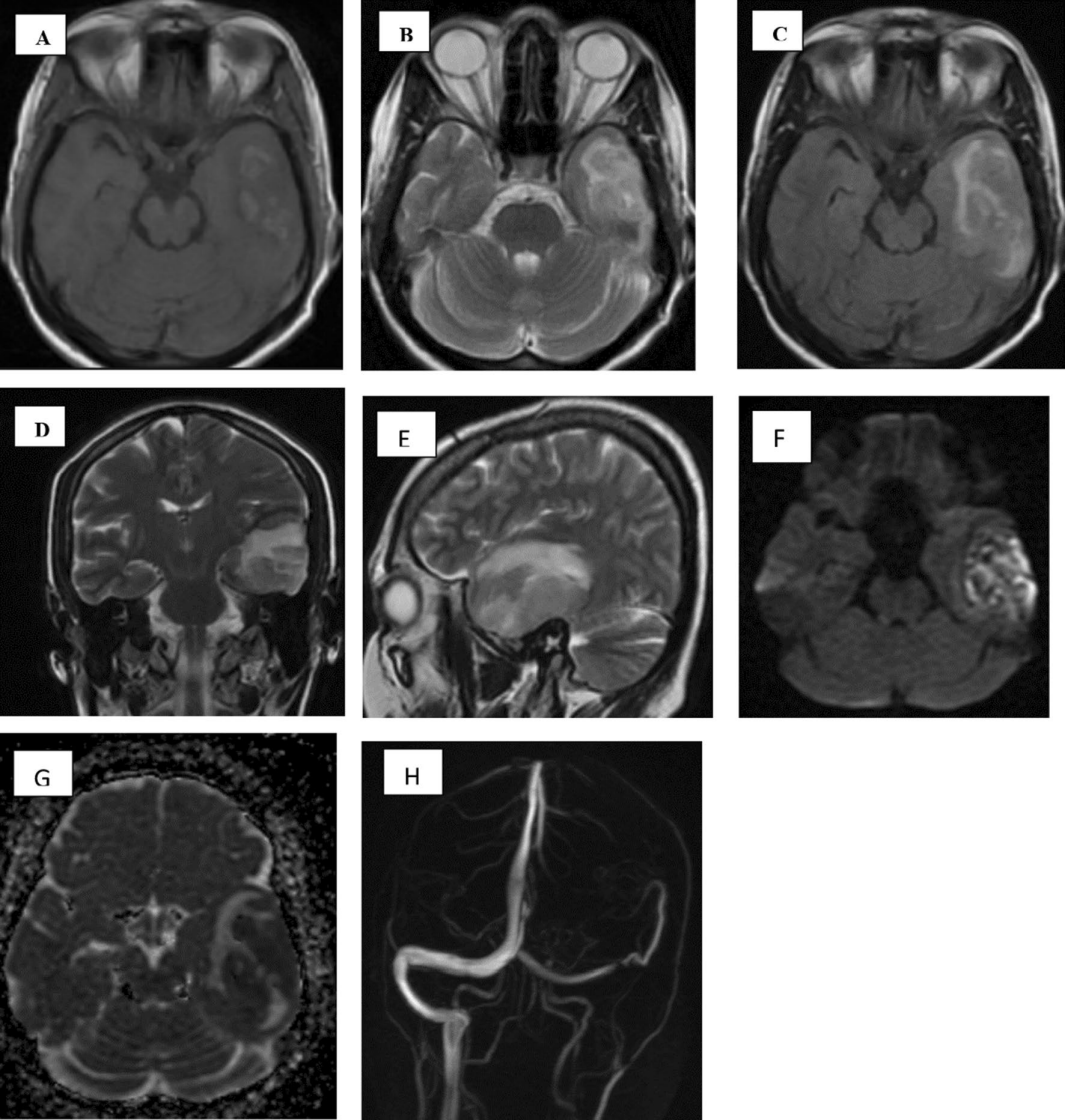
A 63 years-old infected man with COVID-19, presented by blurred vision and vomiting 14 days after initial symptoms, high D-dimer level, MRI study was done and showing left temporal area of altered signal intensity, A axial T1WI shows areas of hyperintensities, B axial T2WI shows heterogenous signal intensities, C axial FLAIR sequence displays high signal, D coronal T2WI, E sagittal T2WI, F DWI has areas of restricted diffusion, G ADC image, MRV in (H, image), shows marked attenuation in left sigmoid, transverse sinus with focal area of signal loss in left transverse sinus and non-visualized left IJV, this is consistent with sinus thrombosis and left temporal haemorrhagic infarction
